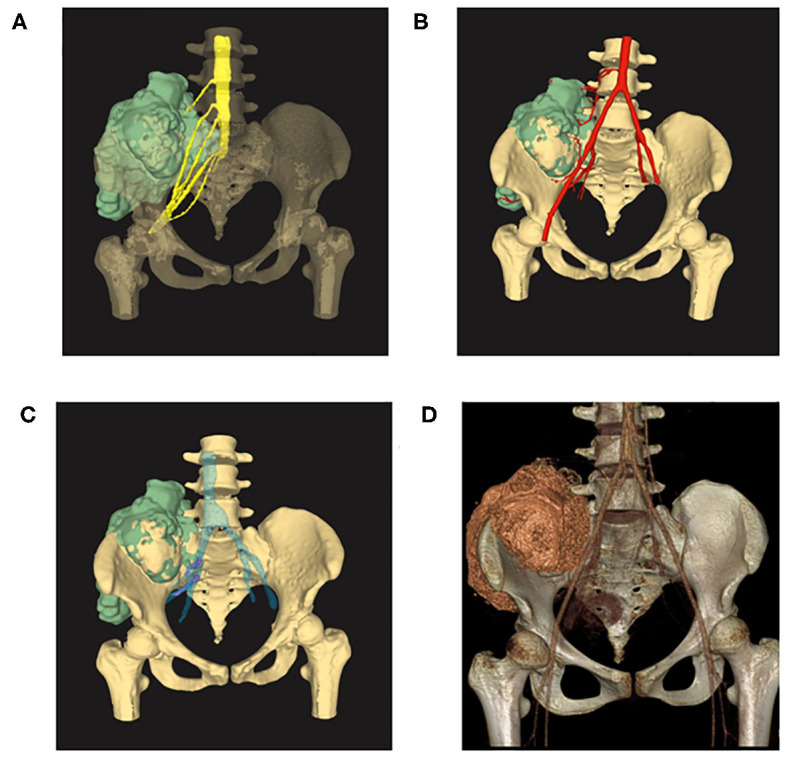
(A) 3-D model reconstructed with AI-assisted CT/MRI image fusion technique. Note that the tumor size was bigger and margins are better shown in this model comparing with the one with solely CT image data. (B) Image fusion data model showing tumor together with adjacent arteries. (C) Image fusion model showing tumor together with adjacent veins. Note that emboli were shown in purple within the veins. (D) 3-D model reconstructed with enhanced CT image data showing tumor of the right ilium extending to the sacrum. Note that the tumor did not invade L5 vertebra in this model.
